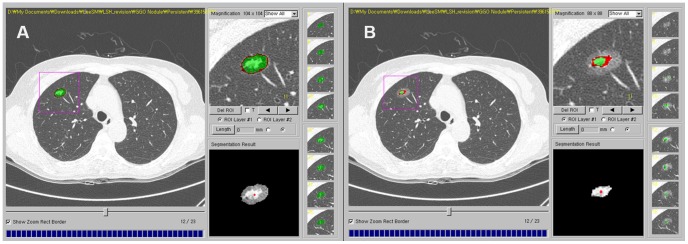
Transverse thin-section CT image showing manual segmentation of a part-solid nodule (PSN). Segmentation of the PSN was manually conducted using an in-house software program and texture features of the nodules were automatically extracted and calculated by the program. One radiologist segmented the outer boundary of the whole PSN (A) and inner solid portion boundary (B).
